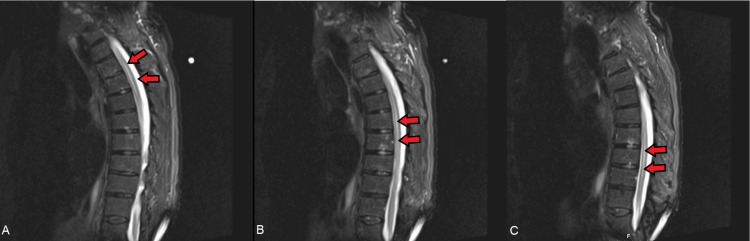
MRI of the thoracic spine (Patient #1). (A) Upper thoracic spine. (B) Middle/lower thoracic spine. (C) Lower thoracic spine. There is diffuse central T2 lengthening throughout the thoracic cord at all segments consistent with transverse myelitis.
